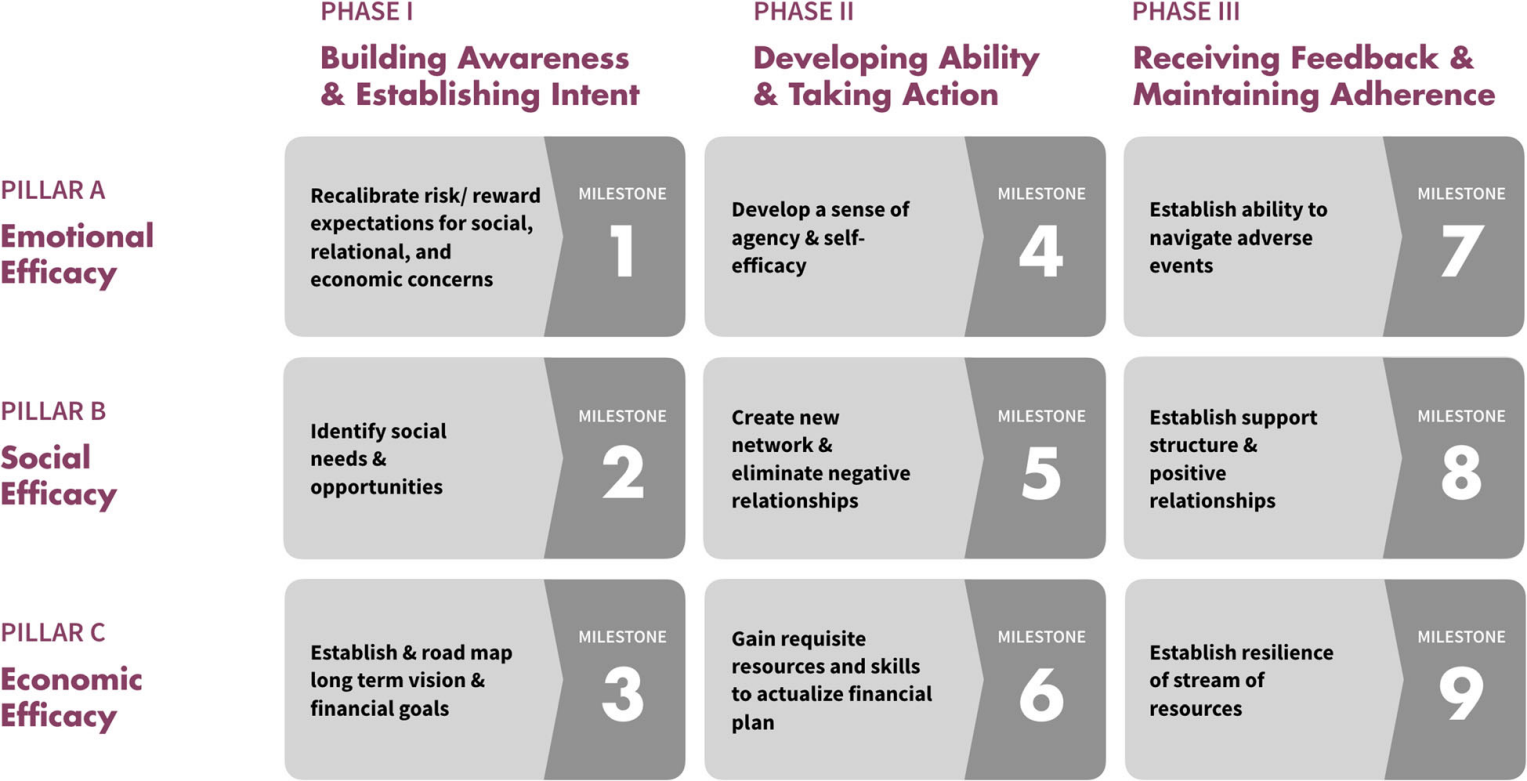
Framework for CT programs for AGYW. 3X3 framework showing pillars and phases of cash transfer program, with milestones for each pillar in each phase
